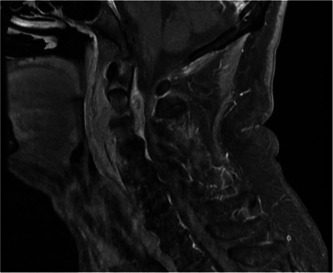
Magnetic resonance imaging cervical spine with contrast demonstrating C2‐3 discitis/osteomyelitis with epidural phlegmon and abscess causing spinal canal narrowing. A small focus of gas in the prevertebral phlegmon raises concern for fistulous communication with the oropharynx.
